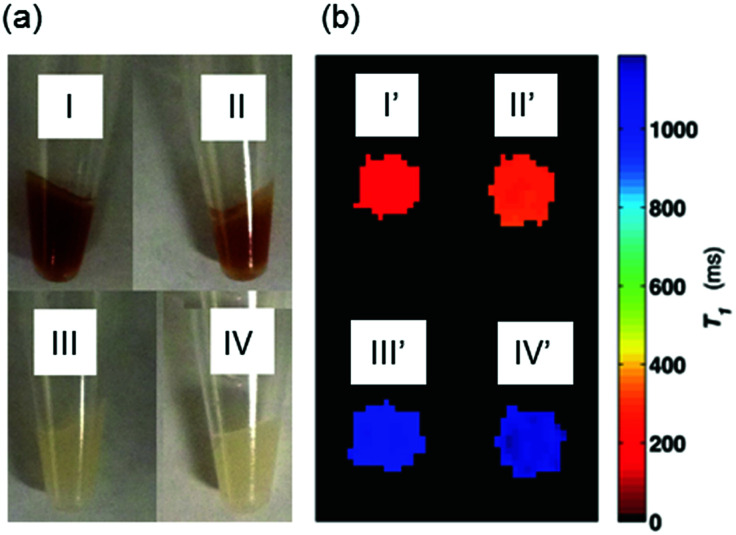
(a) Photograph of cell pellets: (I) MnAMP (II) MnAMP with 5 h in fresh media (III) MnTCP (IV) unlabeled; (b) *T*_1_ maps of the cell pellets estimated from IR-FSE MR images: (I′) MnAMP (II′) MnAMP with 5 h in fresh media (III′) MnTCP (IV′) unlabeled, done at 3 T, room temperature.
